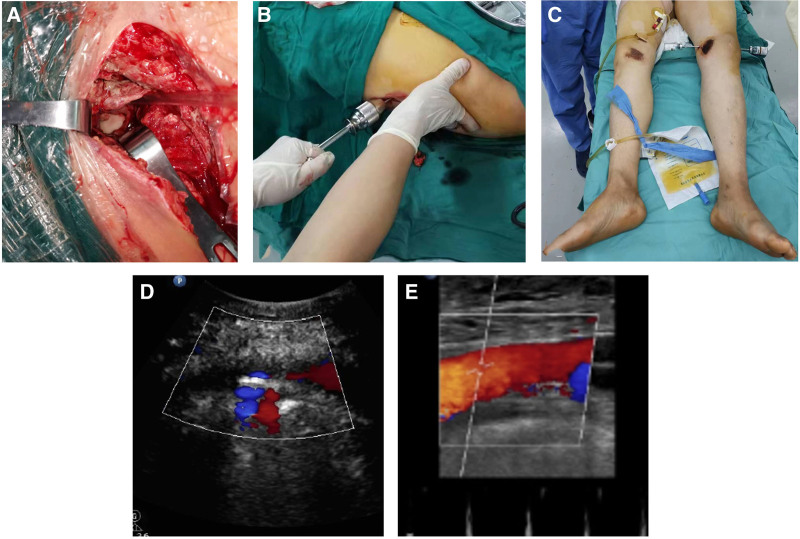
(A) Exploration of the wound revealed a dislocated femoral head with a severe infection of the femoral vascular sheath. (B) Schanz nail was used to assist reduction intraoperatively. (C) The patient’s left lower limb external rotation deformity was corrected after reduction. (D) The intraoperative Doppler ultrasound image showed a lumen-filling defect in the left common femoral artery at the suture. (E) Recovery of luminal blood flow after incision and removal of the thrombus.
